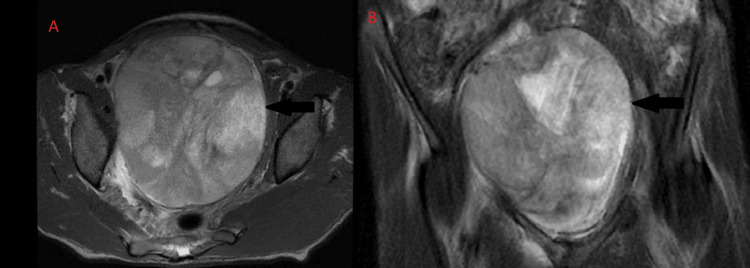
Transverse (panel A) and coronal view (panel B) of the MRI The black arrow points to the mass.
